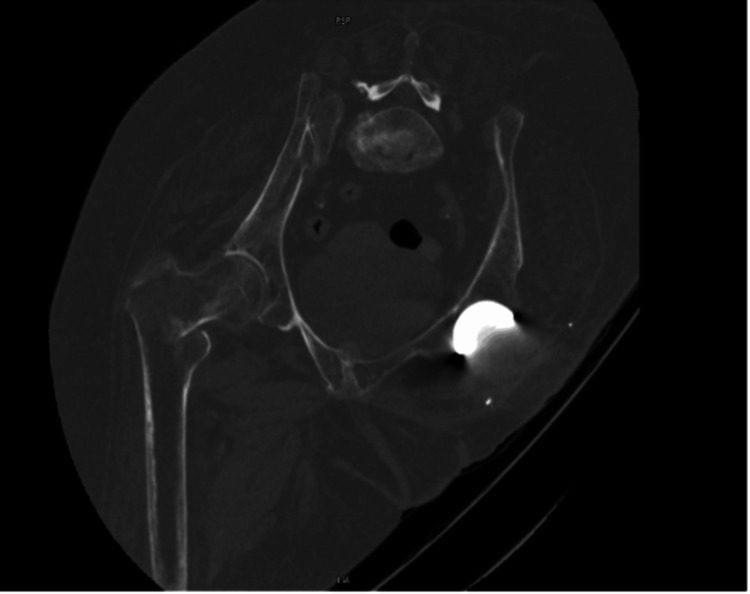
Preoperative CT showing a comminuted intertrochanteric fracture
